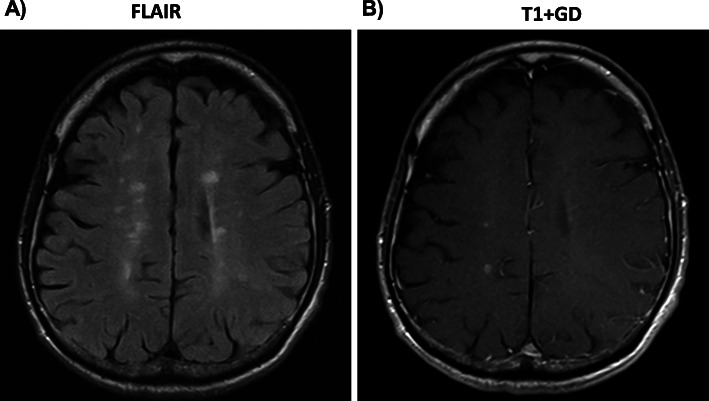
MRI imaging of patient 2 displaying disease activity. a FLAIR and b T1 weighted MRI with application of gadolinium at day 300 after start of ATRA revealed multiple gadolinium-enhancing lesions
